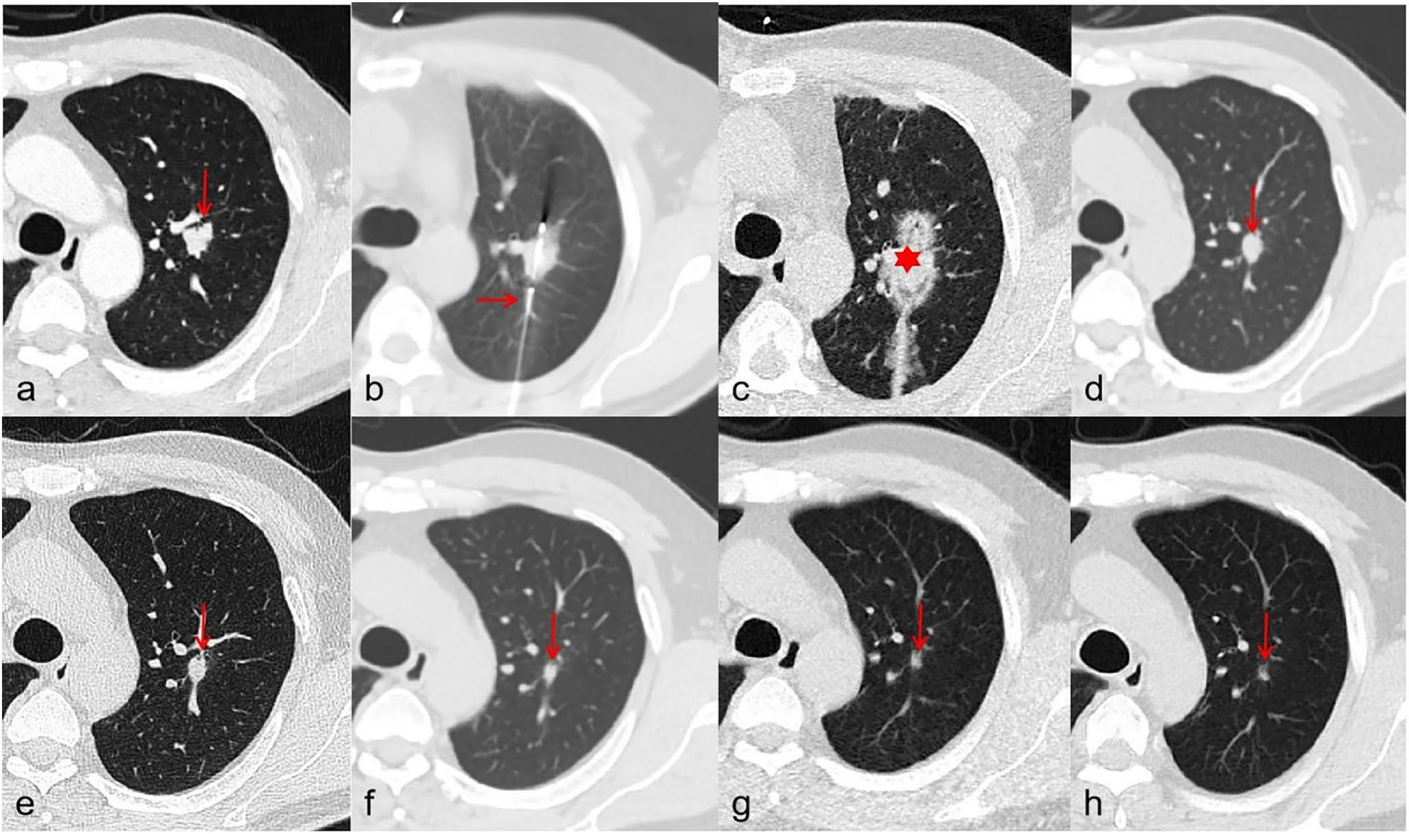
A 43‐year‐old male with a solid nodule which was confirmed to be renal carcinoma with lung metastasis presented a percutaneous cryoablation in the left upper lobe. (a) Before the operation, he accepted a HRCT examination to locate the lesion (arrow). (b) CT scan showing a coaxial introducer needle (arrow) which was placed abutting the lesion. (c) In the CT scan immediately after cryoablation, the ablated nodule presented an “ice ball”—a circular ground‐glass opacity (star). (d–h) The follow‐up CT scans performed 1, 3, 6, 12 and 24 months, respectively, after cryoablation, without tumor recurrence.
